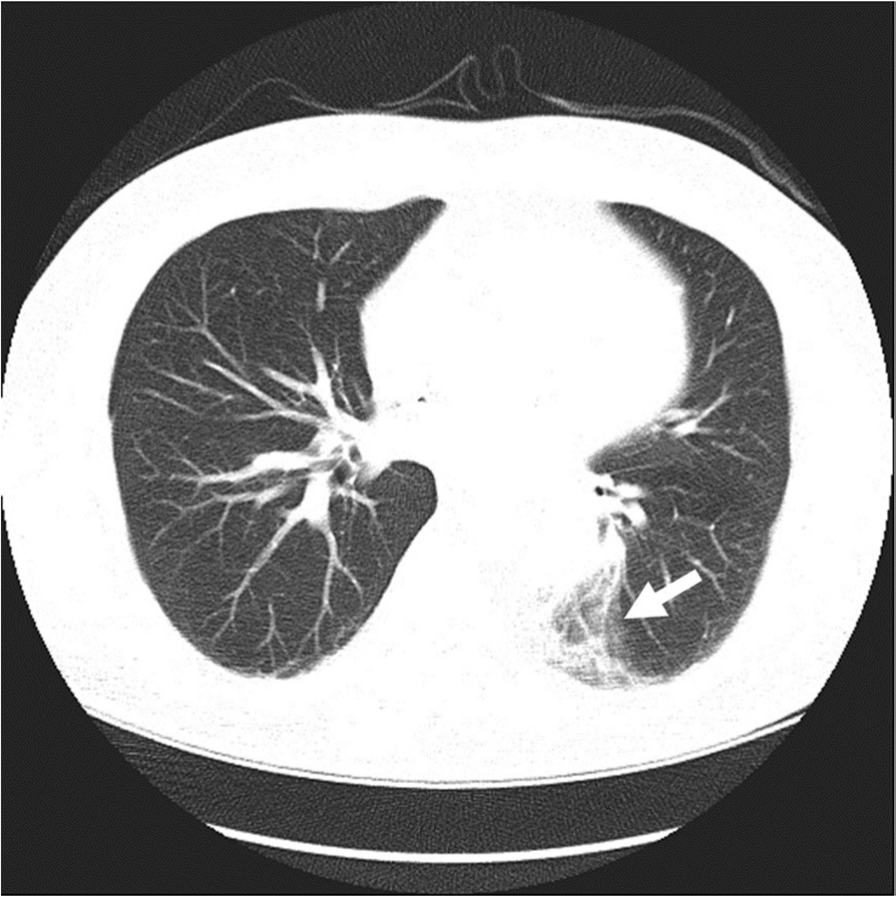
Spiral chest CT depicted a consolidation in posterior aspect of left lung
